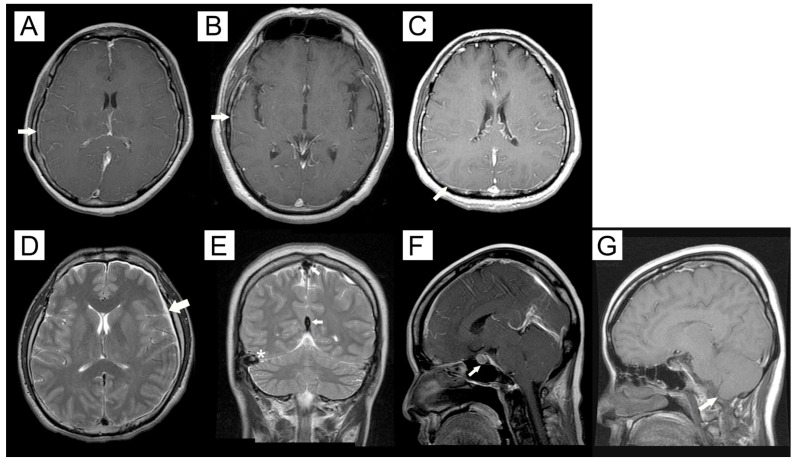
MRI findings of intracranial hypotension. Panels (A–C) were T1-weighted images with contrast enhancement (arrows). Panel (A) revealed generalized enhancement of the meninges, while panel (B) showed partial enhancement of the frontotemporal region, and panel (C) showed partial enhancement of the occipital region. Panel (D): subdural fluid accumulation on the left hemisphere (arrow) on T2-weighted image. Panel (E): engorgement of the superior sagittal sinus (arrows) and transverse sinus (asterisks) on T2-weighted image. Panel (F): T1-weighted image with contrast enhancement demonstrates pituitary hyperemia (arrow). Panel (G): T1-weighted image without contrast shows brain sagging with cerebellar tonsil herniation (arrow).
